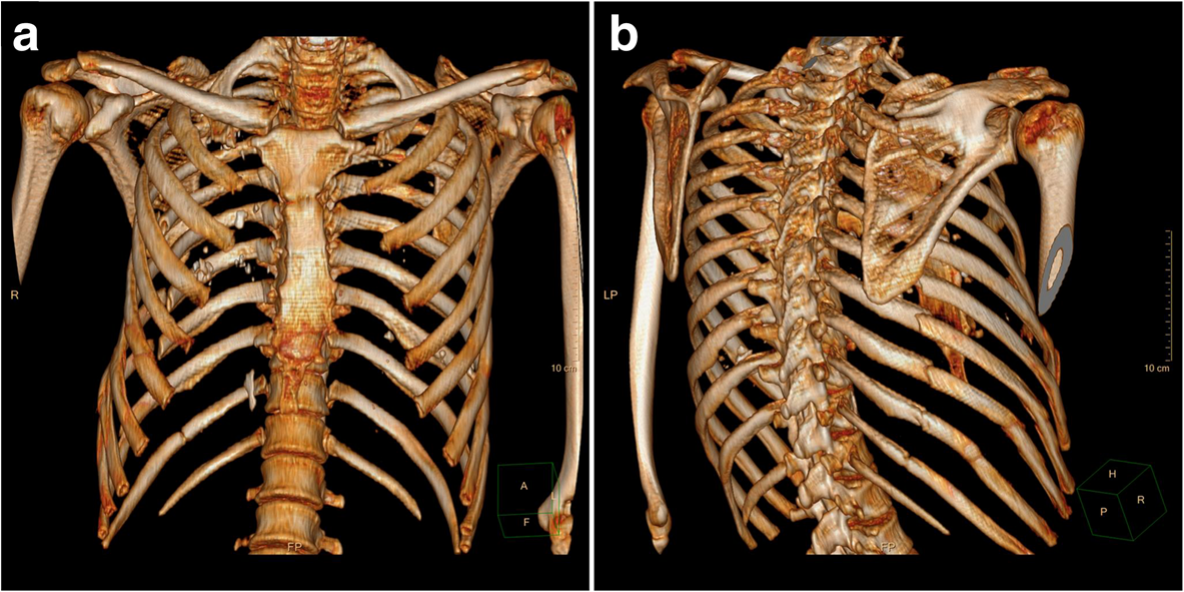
Preoperative three-dimensional reconstruction of the injuries from computed tomographic imaging. Three-dimensional chest computed tomography (a) anterior and (b) posterior reconstructions in a 41-year-old man who sustained fractures to ribs 5–11 on the right posterior side as a result of a traffic accident
